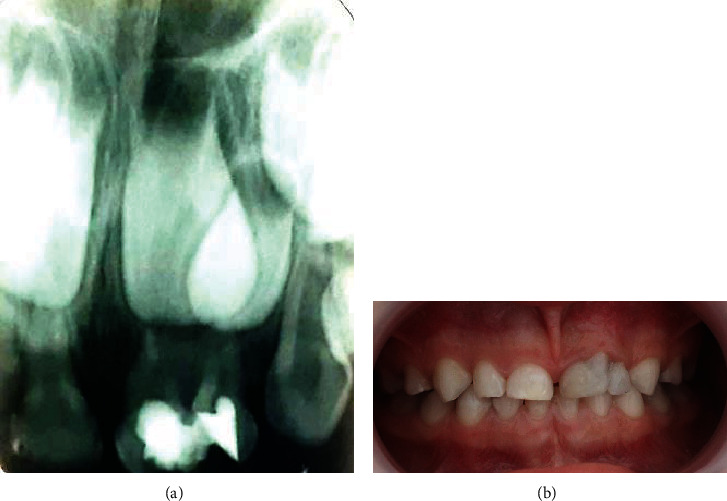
Treatment of teeth 61 and 62. Periapical radiograph showing pulpotomy (a). Coronal restoration (b).
